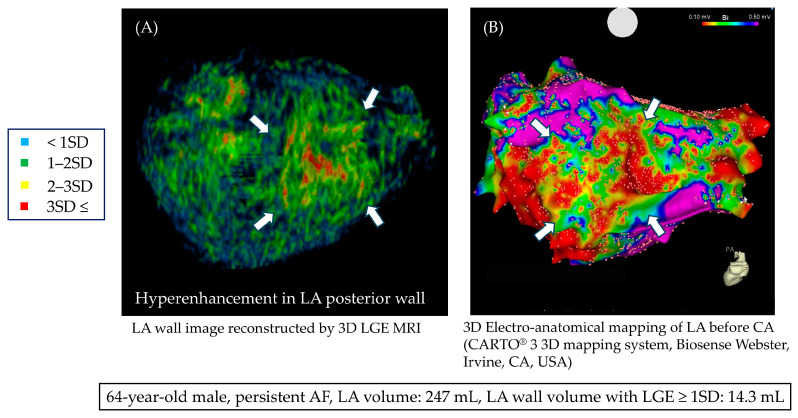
Three-dimensional LGE MRI in a case of persistent AF; (A) LGE MRI shows an area of enhancement on the posterior wall (white arrow). (B) In the 3D voltage map during catheter ablation, a low-voltage area was observed at the site corresponding to the enhancement seen in the LGE MRI.
